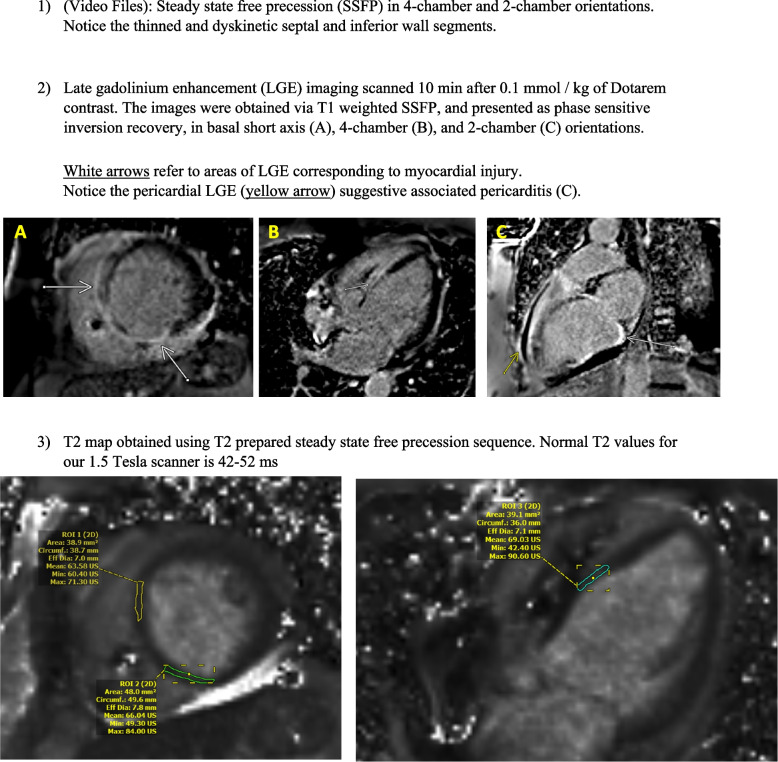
Cardiac magnetic resonance imaging findings. 1) (Video Files): Steady state free precession (SSFP) in 4-chamber and 2-chamber orientations. Notice the thinned and dyskinetic septal and inferior wall segments. 2) Late gadolinium enhancement (LGE) imaging scanned 10 min after 0.1 mmol / kg of Dotarem contrast. The images were obtained via T1 weighted SSFP, and presented as phase sensitive inversion recovery, in basal short axis (A), 4-chamber (B), and 2-chamber (C) orientations. White arrows refer to areas of LGE corresponding to myocardial injury. Notice the pericardial LGE (yellow arrow) suggestive associated pericarditis (C). 3) T2 map obtained using T2 prepared steady state free precession sequence. Normal T2 values for our 1.5 Tesla scanner is 42–52 ms
